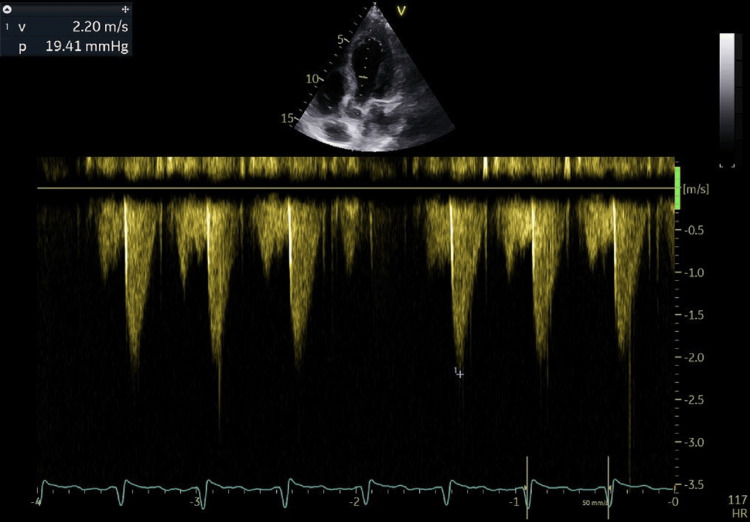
TTE showing the absence of an LV intracavitary gradient following exercise TTE: trans-thoracic echocardiography, LV: left ventricular
